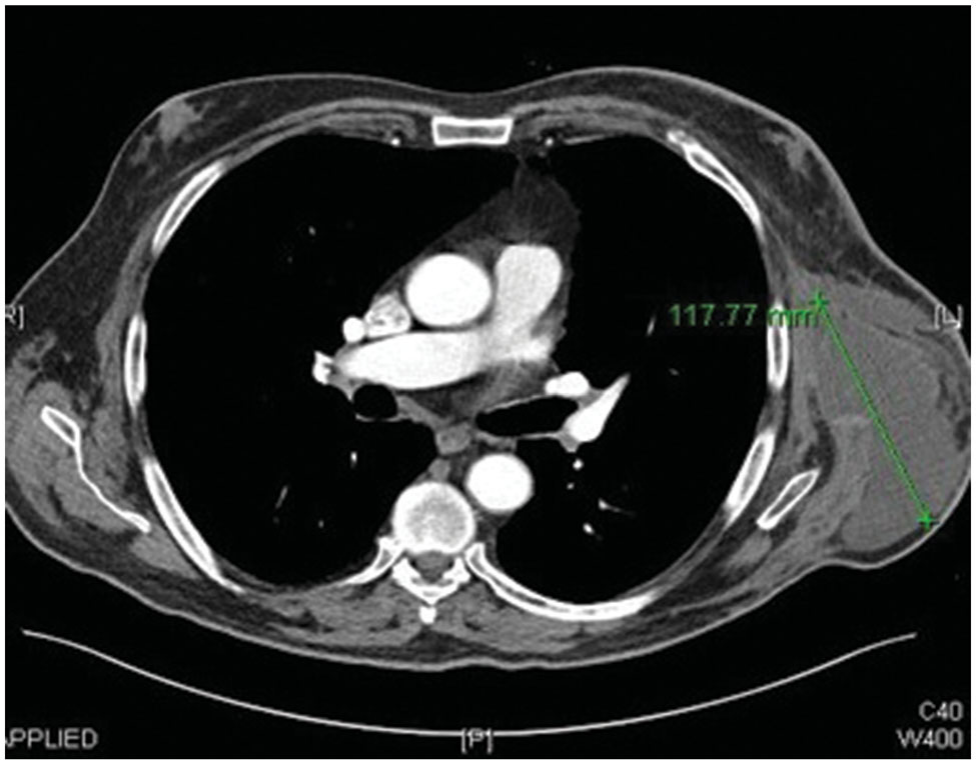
CT scan image 1-month post-resection of chronic seroma capsule and free ALT-flap inset.
